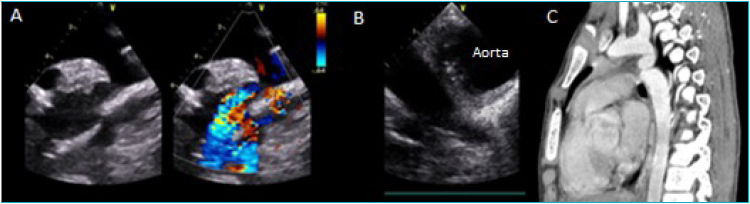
A. Transthoracic echocardiogram (TTE) Suprasternal view: aortic arch with an area of coarctation and post-stenotic dilation.B. Transesophageal echocardiogram (TEE) short-axis view (0°) of the descending aorta at the post-coarctation mid-esophageal level, where an image of vegetation can be seen. C. Angiotomography showing aortic coarctation.
